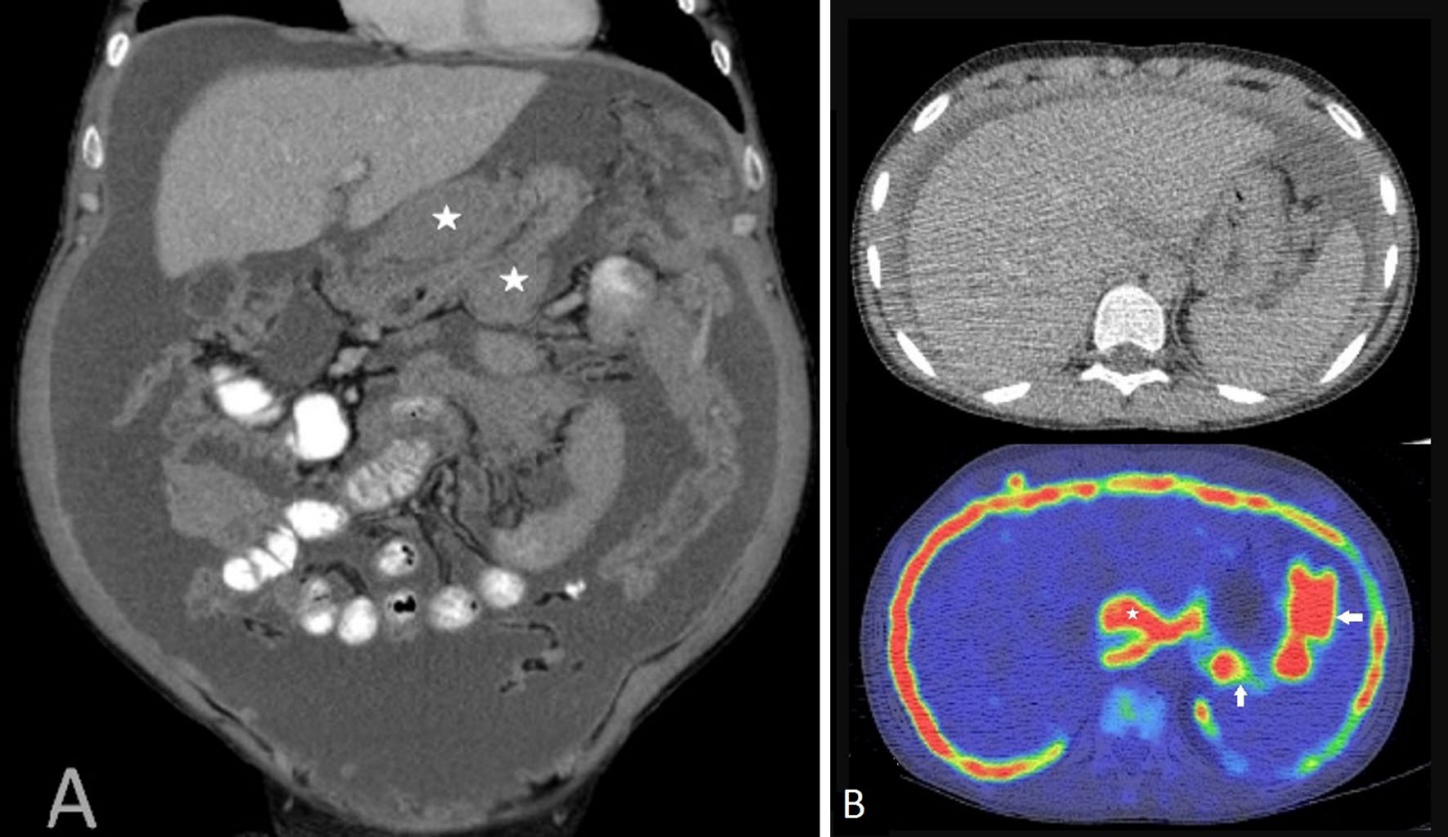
a, b Images of two patients with epithelioid malignant peritoneal mesothelioma showing disease in the epigastric region. a Coronal CT with extensive peri-gastric disease (*) seen as soft tissue masses around the stomach. Also note the tethered mesentery. b Axial PET-CT images showing FDG-avid disease in the lesser omentum, lesser sac, porta (*) and peri-gastric region (arrows)
